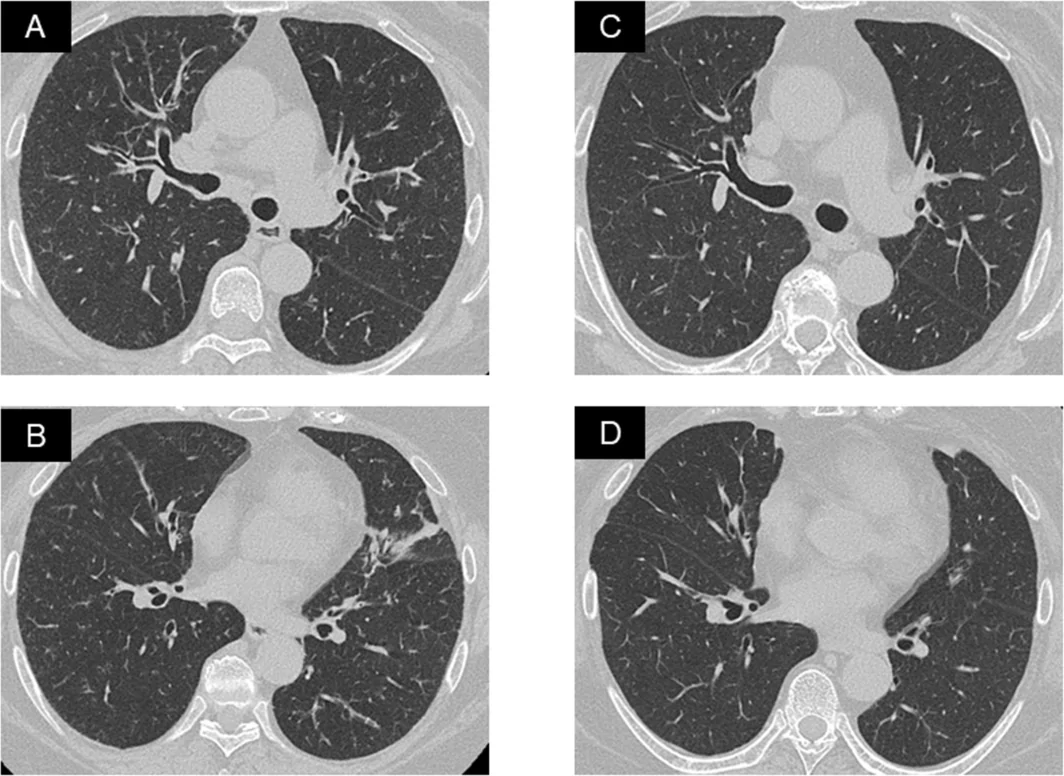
Chest computed tomography before and after corticosteroid therapy. (A, B) Pretreatment chest computed tomography demonstrating diffuse bilateral centrilobular nodules and bronchial wall thickening. (C, D) Follow‐up computed tomography after corticosteroid therapy showing marked improvement of the centrilobular nodules and airway lesions.
